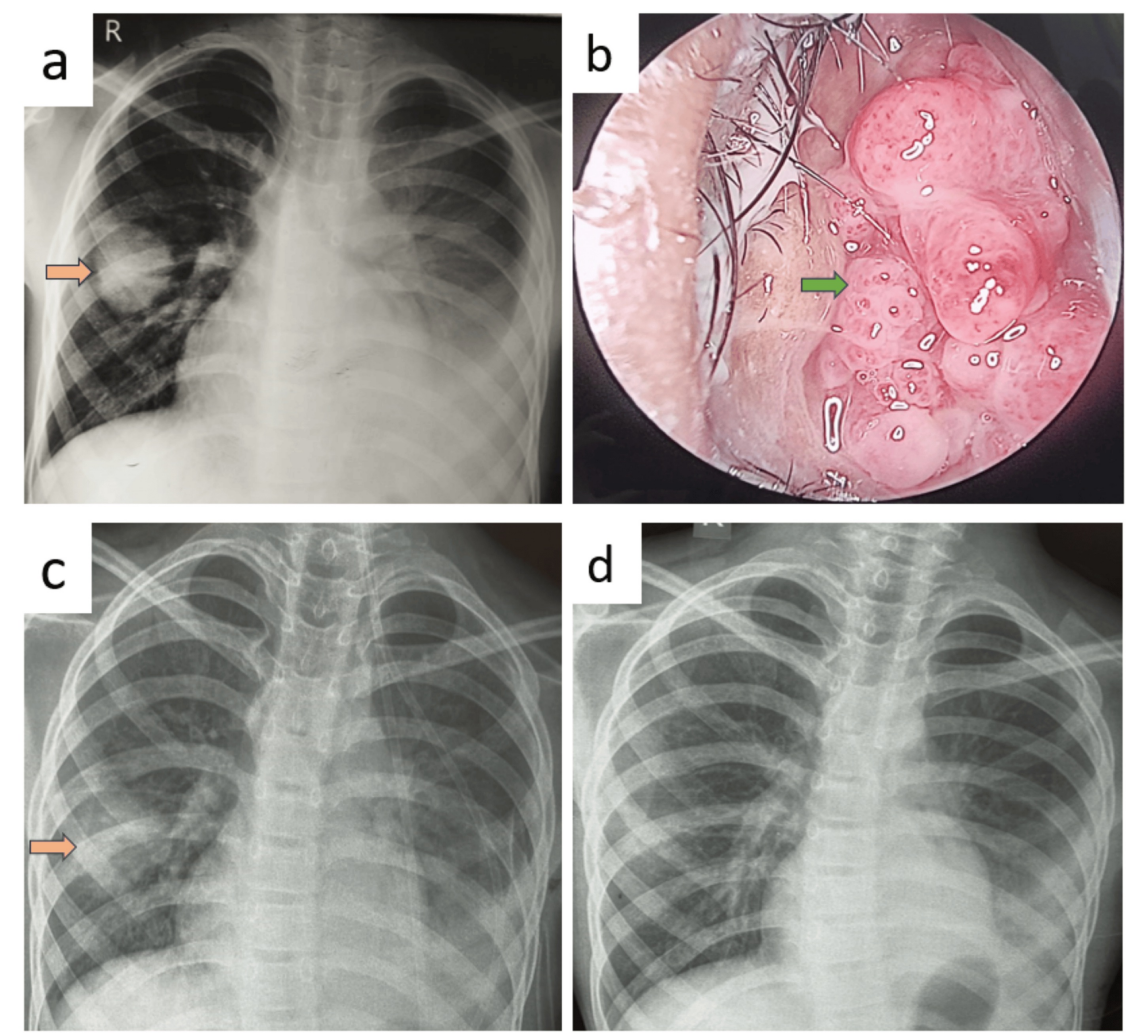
Chest X-ray and anterior rhinoscopy images of patient E before and after treatment. (a) Chest X-ray findings included well-defined rounded opacity in the right mid-lung zone (marked by a brown arrow) and left-sided pleural effusion. (b) Anterior rhinoscopy revealed left nasal polyposis marked by a green arrow. (c) Chest X-ray after a month showed a decrease in the density of the lesion marked by a brown arrow. (d) Three months later, chest X-ray showed near complete resolution of bilateral lesion.
